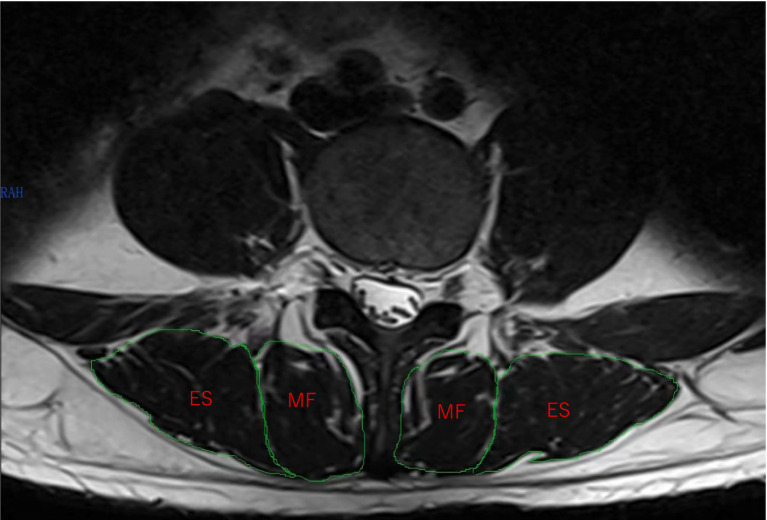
Cross-sectional magnetic resonance imaging of the fourth lumbar spine. MF, multifidus; ES, erector spinae.
